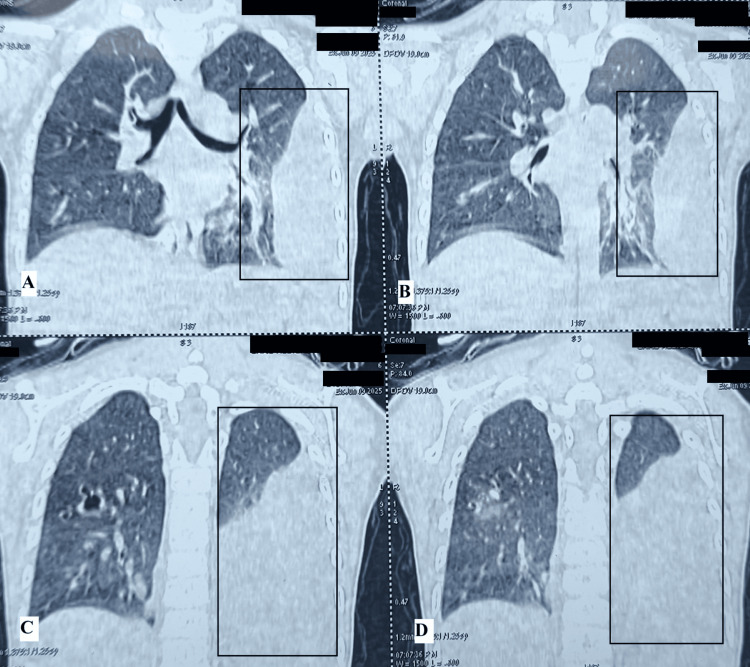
Sagittal view of baseline high-resolution computed tomography thorax shows left-sided pleural effusion, passive lung collapse, bronchiectasis, and ground-glass opacities in the highlighted box.
